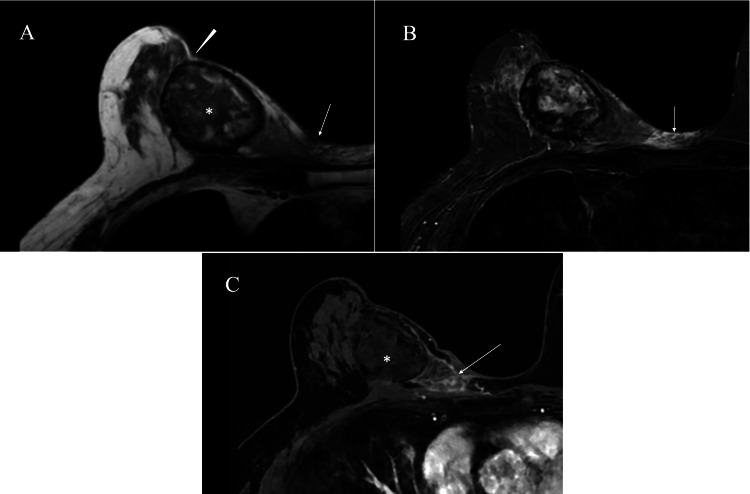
MRI findings (A) T1-weighted images showed an RFA nodule (asterisk), presumed adhesion between the RFA nodule and the overlying skin (arrowhead), and low signals at the skin (arrow) due to cellulitis. (B) MRI of the skin just above the sternum showed high signals (arrow) on T2-weighted images, suggesting inflammation, i.e., cellulitis. (C) Dynamic studies showed enhancement in the areas (arrow) medial to the RFA nodule, presumably due to cellulitis. MRI: magnetic resonance imaging, RFA: radiofrequency ablation
